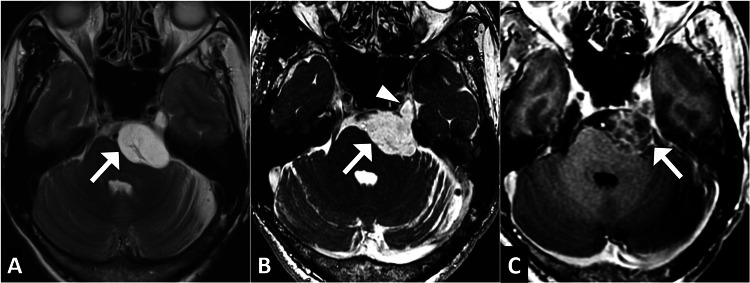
Axial brain MRI images in T2-weighted image (A), CISS image (B), and T1-post contrast image (C) showing a well-defined lesion (arrow) at the left cerebellopontine angle extending into the left Meckel's cave (arrowhead), suggestive of trigeminal schwannoma CISS: constructive interference in steady state image, MRI: magnetic resonance imaging
